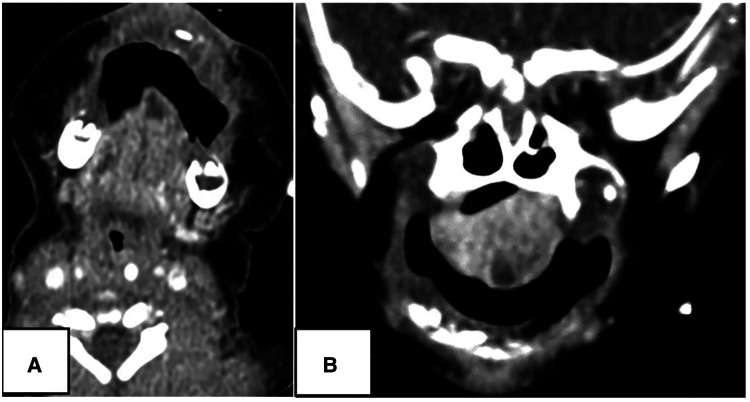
A 6-hour-old Male. CT showed a 1.0-cm sized cystic lesion in the ventral tip of tongue (white arrow): (A) contrast-enhanced axial scan; (B) coronal scan.
